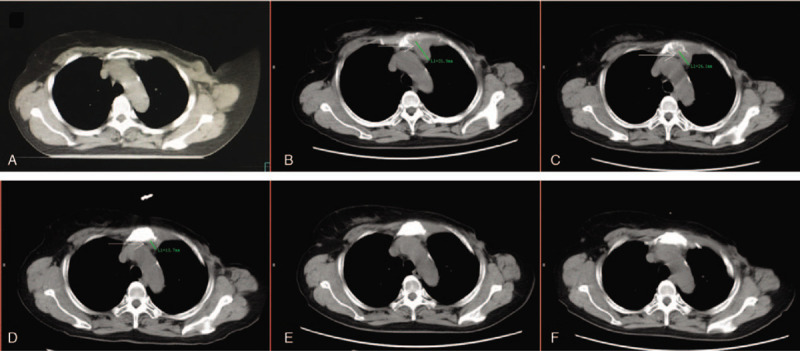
Continuous shrinkage of the internal mammary lymph nodes (IMN) metastases and the involved bone repair, which are marked with arrows. (A) In planning computed tomography (CT) prior to the first course of radiation therapy. (B) CT prior to the second course of reirradiation therapy. (C) In control CT at 21st fraction of reirradiation. (D) Follow-up CT at 1 month after reirradiation therapy. (E) Follow-up CT at 3 months after reirradiation therapy. (F) Follow-up CT at 6 months after reirradiation therapy.
